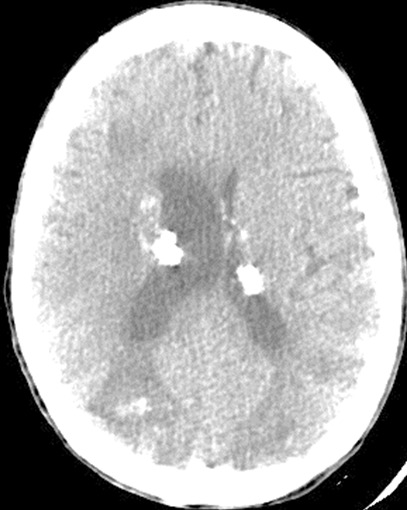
Axial unenhanced CT scan of the brain shows numerous calcified sub ependymal nodules
